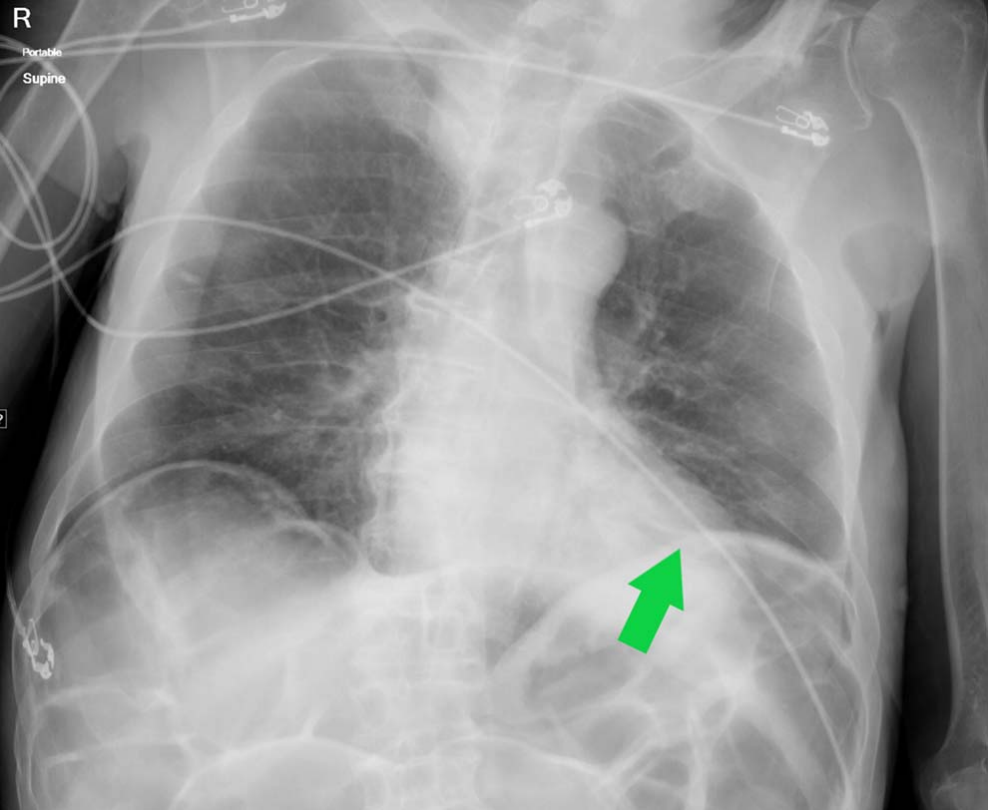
Portable supine anteroposterior radiograph of an 80-year-old male shows unilateral discoid (arrow) opacity along the left hemidiaphragm, typical of subsegmental atelectasis. This pattern was considered atypical and less suspicious for coronavirus disease 2019.
